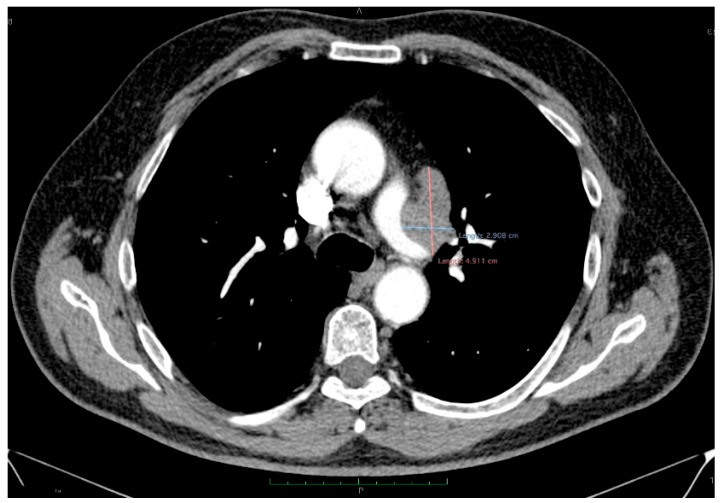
Contrast-enhanced thoracic CT (axial, arterial phase; mediastinal window) demonstrates a lobulated mediastinal mass with heterogeneous enhancement, abutting the right pulmonary artery, measuring approximately 49 × 29 mm in the aortopulmonary window.
